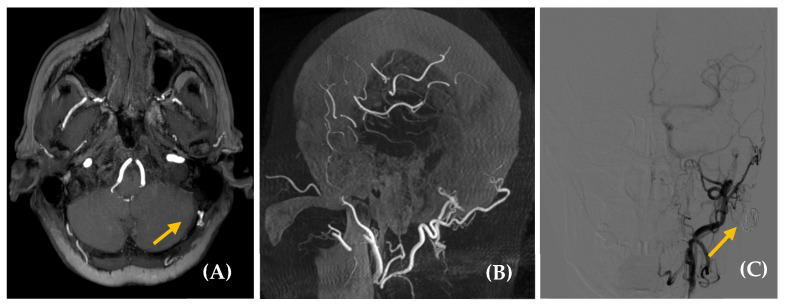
Dural arteriovenous fistula (dAVF) in a 65-year-old woman with a somatosound in the left ear, present for five years. The PT neither disappeared nor changed upon compression of the cervical vascular axis. MR angiography axial plane (image (A)) revealed findings suggestive of a dAVF (yellow arrow). Diagnostic–therapeutic arteriography (images (B), cone bean angio CT MPR in sagittal plane and (C), posteroanterior DSA view with injection of extracellular contrast agents) confirmed the presence of an AVF with arterial supply from the left occipital branch and superficial venous drainage to the left suboccipital region. Based on this finding, the fistula was embolized (yellow arrow, image (C)).
